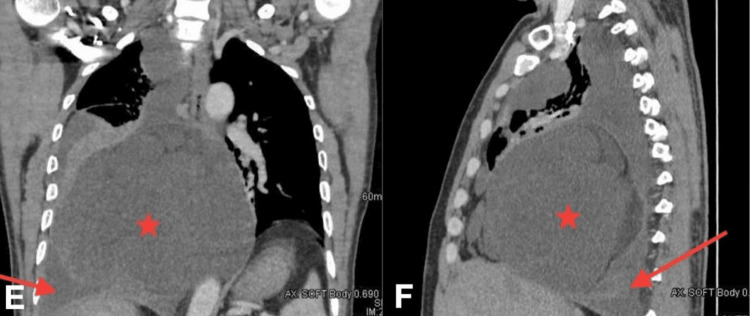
Enhanced coronal (E) and sagittal (F) chest CT scan soft tissue window shows a large well-demarcated mass (star) with peripheral enhancement with the main bulk of it in the right lower thoracic cavity and to a lesser extent in the mediastinum and multiloculated right pleural effusion with thick irregular pleural enhancement and multiple septations (arrow). CT: computed tomography
